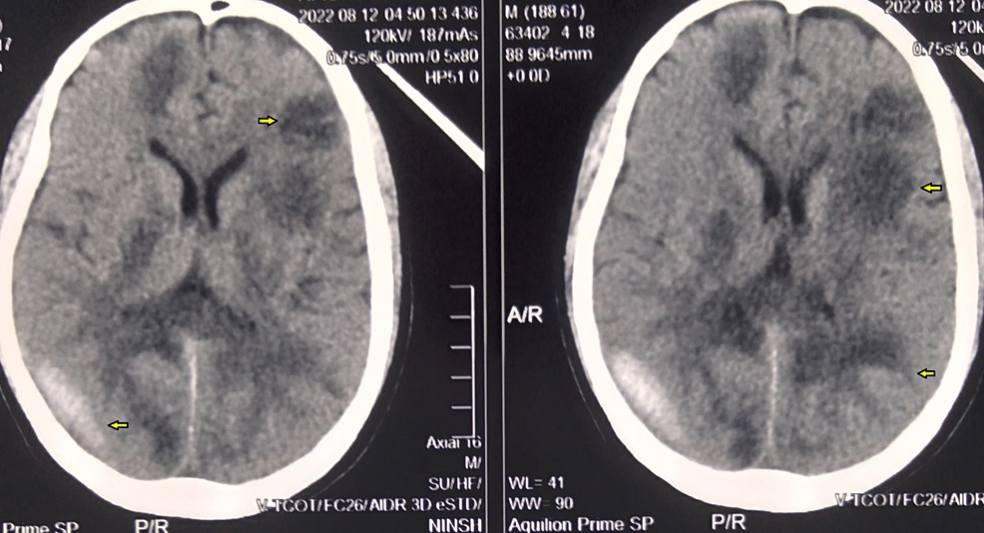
CT scan head (non-contrast} showing multiple mixed-density lesions (yellow arrows) all over the cerebral cortex
